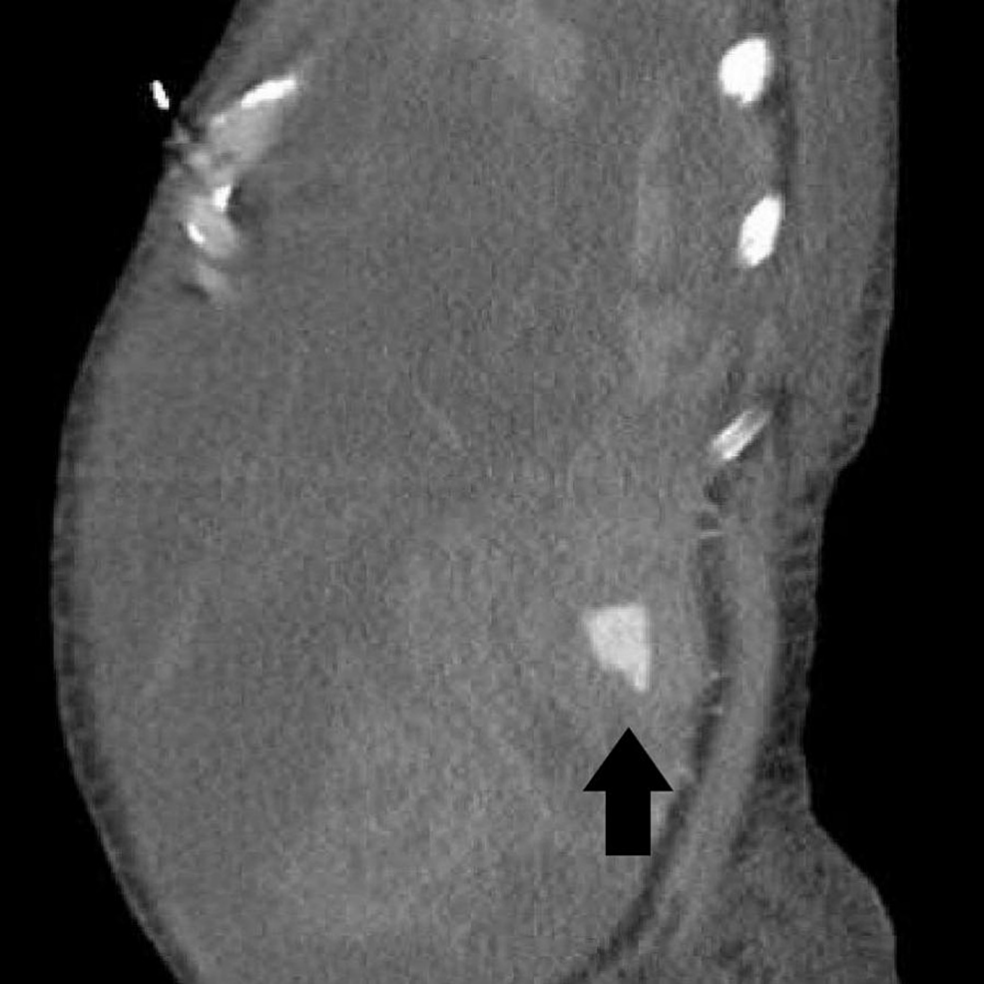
CT with angiography showing hemoperitoneum with hemorrhagic mesenteric varix (black arrow); sagittal view.
